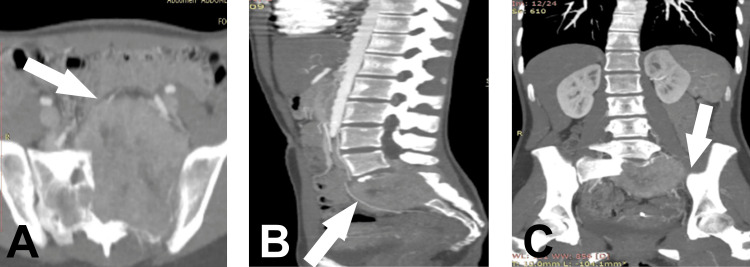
Contrast-enhanced lumbo-pelvic CT scan in (A) axial, (B) sagittal, and (C) coronal views. Note that the extensive tumoral mass (arrow) has obliterated the surrounding bony tissue, especially, the left sacroiliac joint with a prominent extension into the abdominal cavity.
